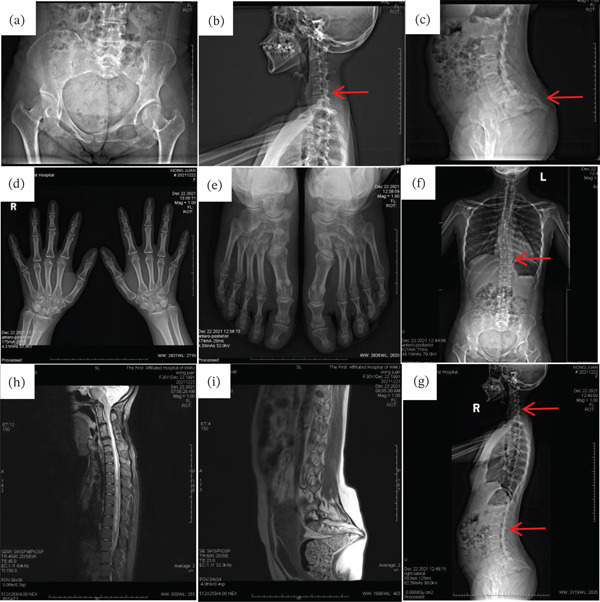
X‐ray and MRI of mother of patient. (a) Asymmetry hip. (b) Vertebral fusion at C2–3, C4–5, and C6–7 levels. (c) Horizontally oriented, hook‐shaped coccyx. (d, e) Absence of carpal and tarsal bone fusion. (f) thoracic scoliosis. (g) Loss of cervical lordosis with excessive lumbar lordosis. Spinal MRI show (h, i) heterogeneous signal intensity in thoracolumbar spinal cord with abnormal sacrococcygeal development, with a horizontally oriented, hook‐shaped coccyx.
